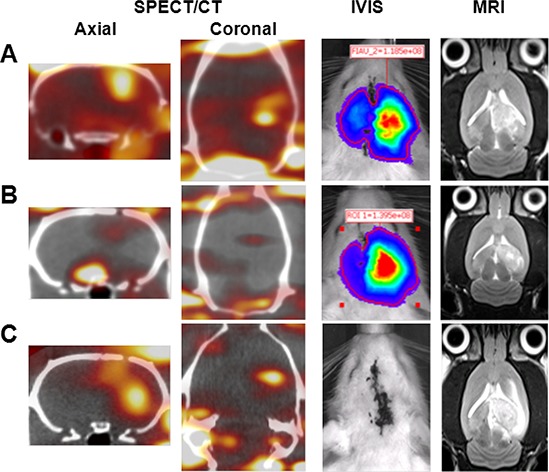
Representative micro-SPECT/CT, bioluminescence, and MR images obtained on day 10 after tumor implantation F98/FGT gliomas with and without FUS-induced BBB disruption are shown in A. and B. respectively. F98 gliomas with BBB disruption are shown in C.
